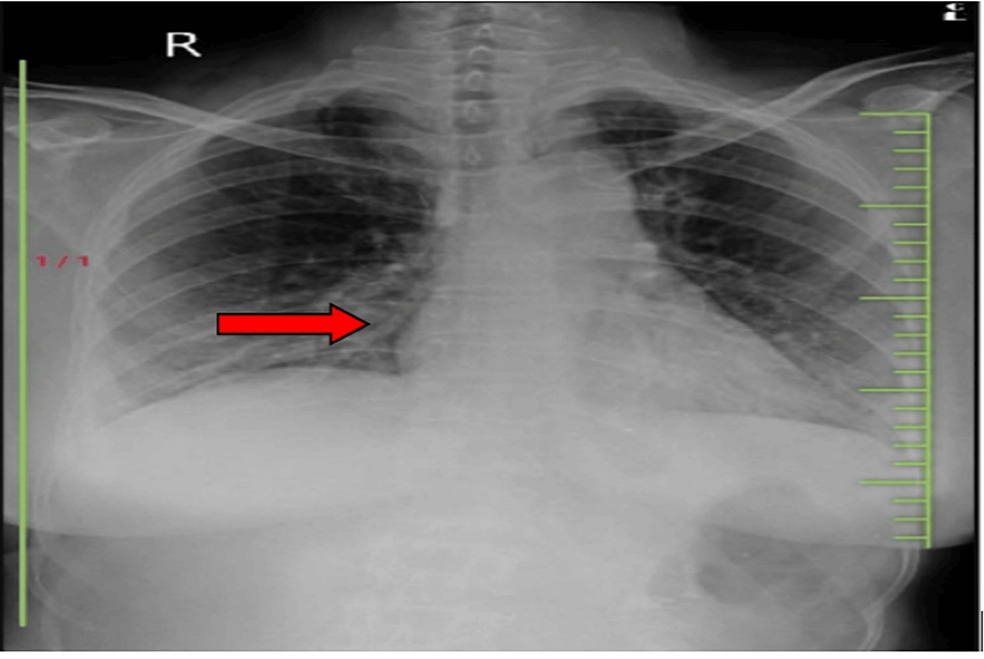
X-ray chest An X-ray revealed heterogeneous opacity on the right middle and lower zones, and prominent broncho vascular marking (red arrow).
